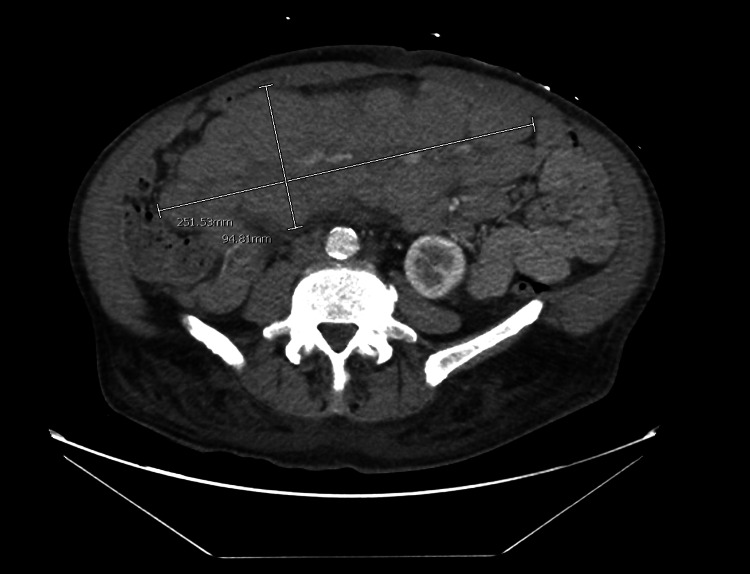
A CT scan of the chest reveals massive confluent lymphadenopathy in the mesentery
